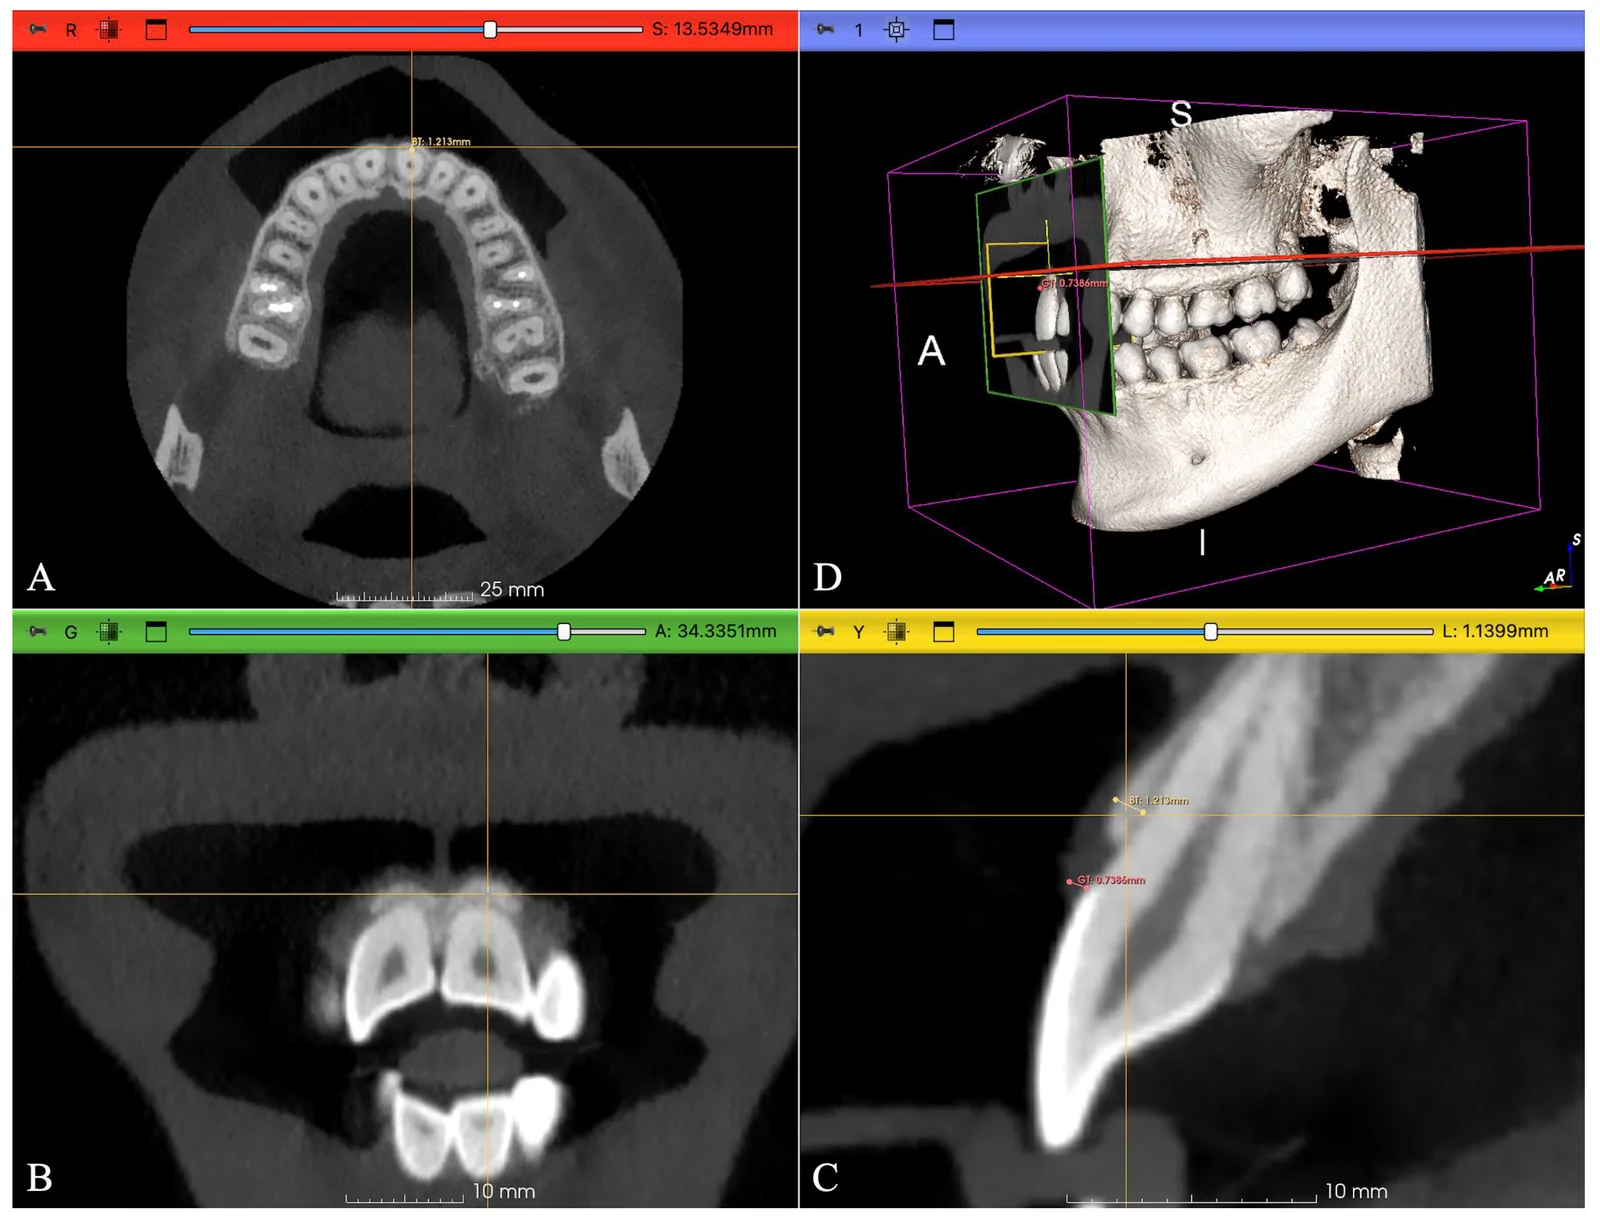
Measurement of gingival thickness and bone thickness on cross-sectional CBCT images. (A) Axial, (B) coronal, and (C) sagittal planes, and (D) three-dimensional (3D) volume-rendered reconstruction (A: anterior, S: superior, I: inferior).
